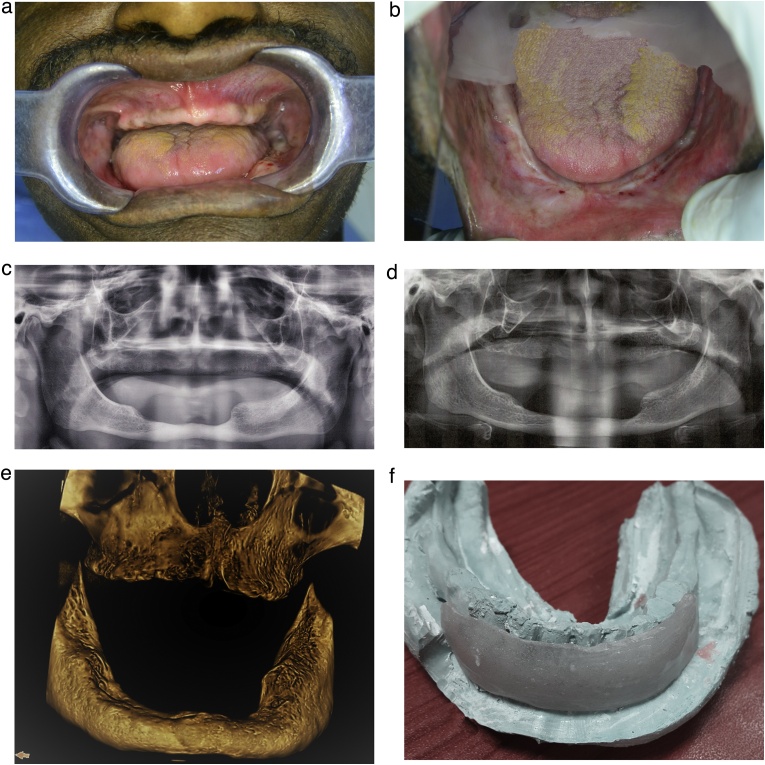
The patient’s clinical presentation. (a) The intraoral view presenting a severely resorbed maxillary edentulous ridge. (b) The intraoral view presenting an anterior marginal resected mandibular bone associated with an obliterated sulcus. (Image was taken using a mirror). (c) The panoramic radiograph shows hypocellularity in the maxilla and mandible. (d) The preoperative panoramic view of the patient after the first 20 sessions of Hyperbaric oxygen. (e) The preoperative 3D view of the maxilla and mandible using cone-beam computed tomography. (f) A photograph showing a heat-cured acrylic stent to be inserted following vestibuloplasty, to ensure the correct repositioning of the muscles.
